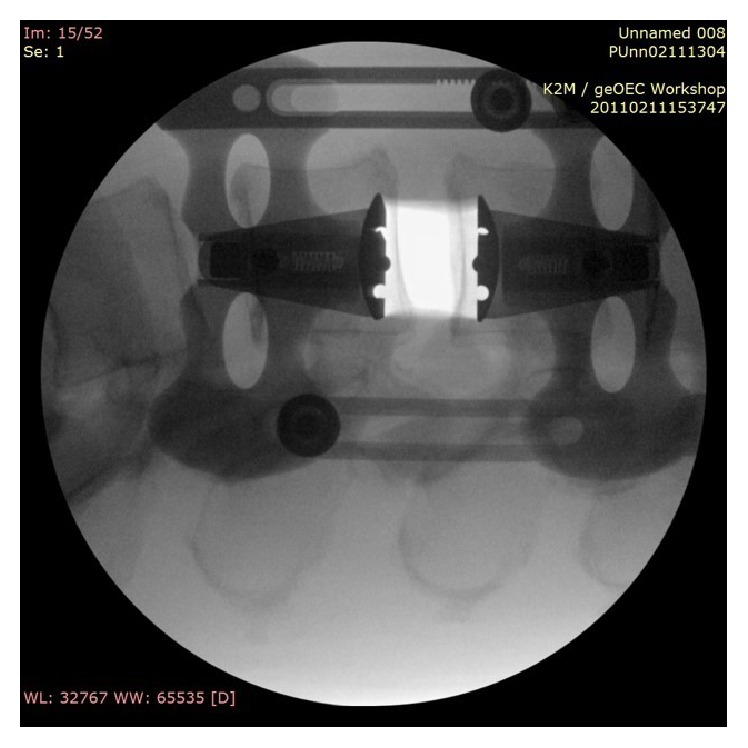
Intraoperative radiograph of RAVINE.
